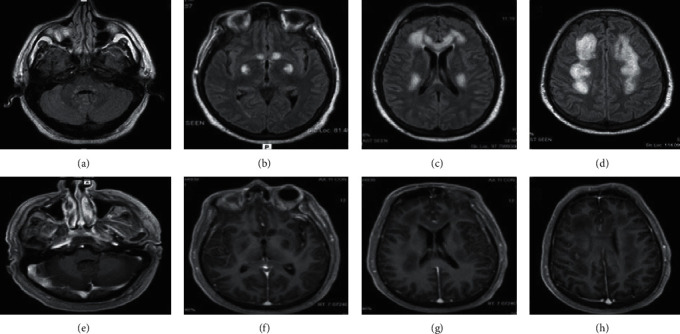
FLAIR brain MRI showing hyperintensity involving peduncles and middle cerebellar peduncles (a). Head of the caudate nucleus and both globus pallidus (b). Corpus callosum and corona radiata (c, d). Gadolinium T1 sequence (e–h) showing focal pathological enhancement in the right parietal lobe (h).
